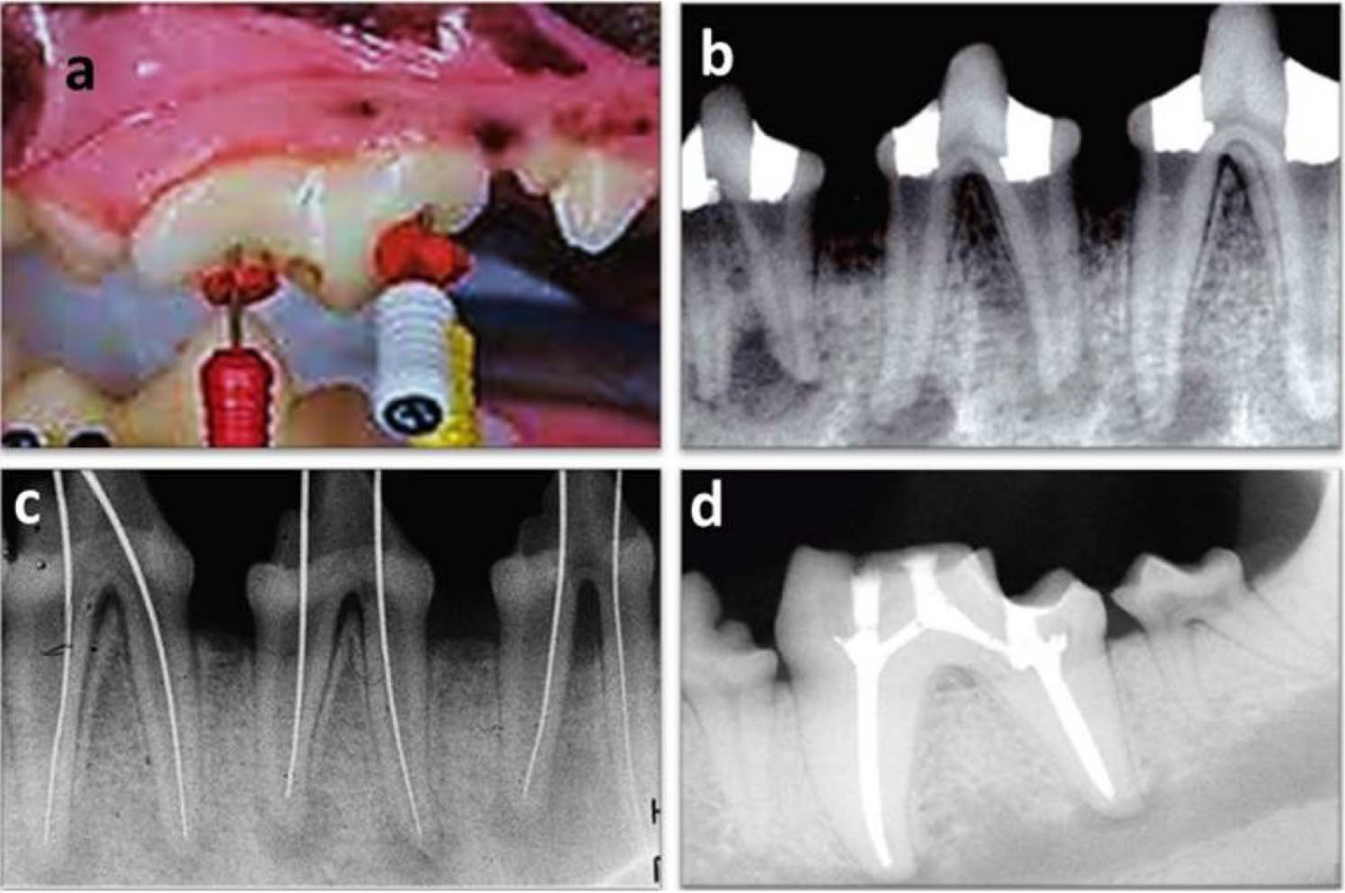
(a) Representative photograph showing preparation of the access cavities. (b) Representative photo-radiograph showing development of periapical pathosis. (c) Representative photo-radiograph showing determination of the working length. (d) Representative photo-radiograph showing obturated root canals.
